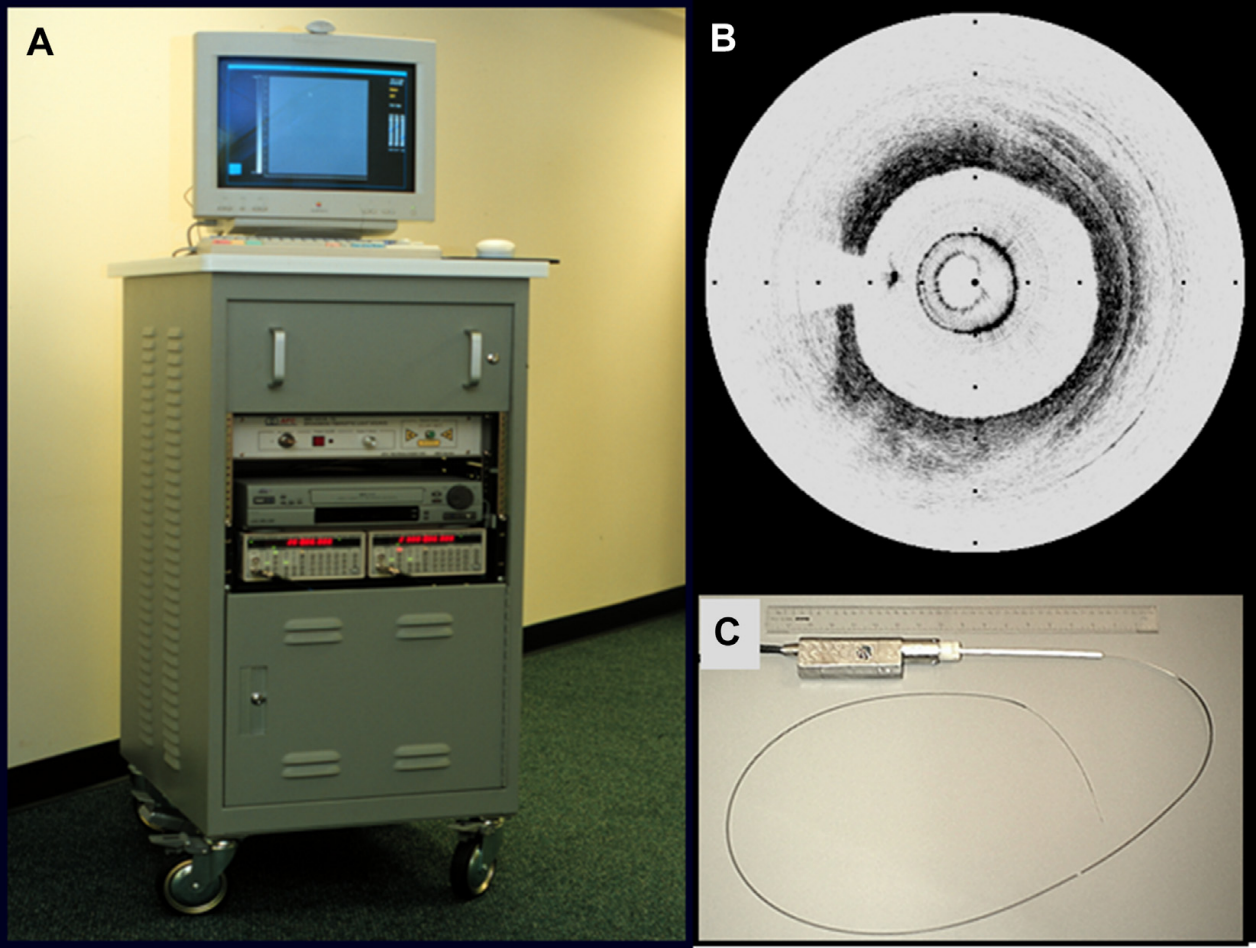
Prototype Console (A) Prototype OCT console built at Wellman Laboratory of Photomedicine, Massachusetts General Hospital. (B) Image was acquired by flushing saline distal to the occlusion balloon. (C) Prototype catheter was built by replacing the transducer core of a commercial intravascular ultrasound catheter with an optical fiber. Reprinted with permission from Yonetsu et al.^87^ OCT = optical coherence tomography.
